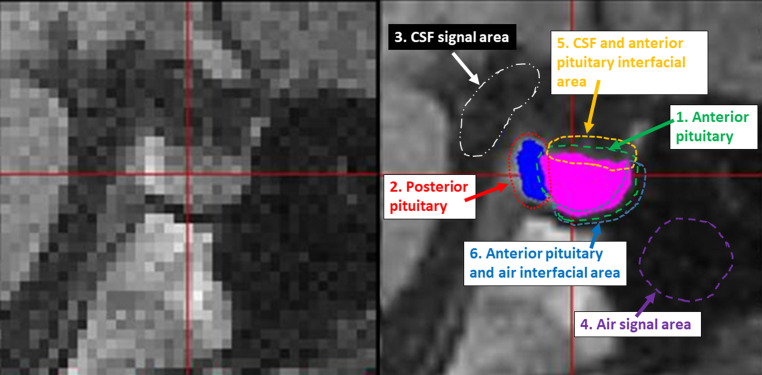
Demonstration of semi-automatic threshold in V3D anterior pituitary volumetry. The *left* shows a T1-weighted mid-sagittal MR image of the pituitary gland. On the *right*, the image is 6‑fold interpolated, and manually drawn ROIs used for generating the anterior pituitary mask are shown. ROIs of anterior (1) and posterior (2) pituitary were sufficiently eroded to remove the exterior signal background before the interior signal was averaged. The signals for CSF (ROI 3), and air space (ROI 4) were directly averaged. The boundary between the anterior pituitary and CSF was determined in ROI 5 by setting the threshold to the average of CSF mean value and anterior pituitary mean value. The boundary between the anterior pituitary and air space was determined in ROI 6 by setting the threshold to the average of air space mean value and anterior pituitary mean value. The boundary between the anterior and posterior pituitary was determined inside the overlapping region of ROIs 1 and 2, with the threshold equal to the average signal intensity of the two glands. The *solid pink and blue areas* represent the final masks used for anterior and posterior pituitary volume calculation
